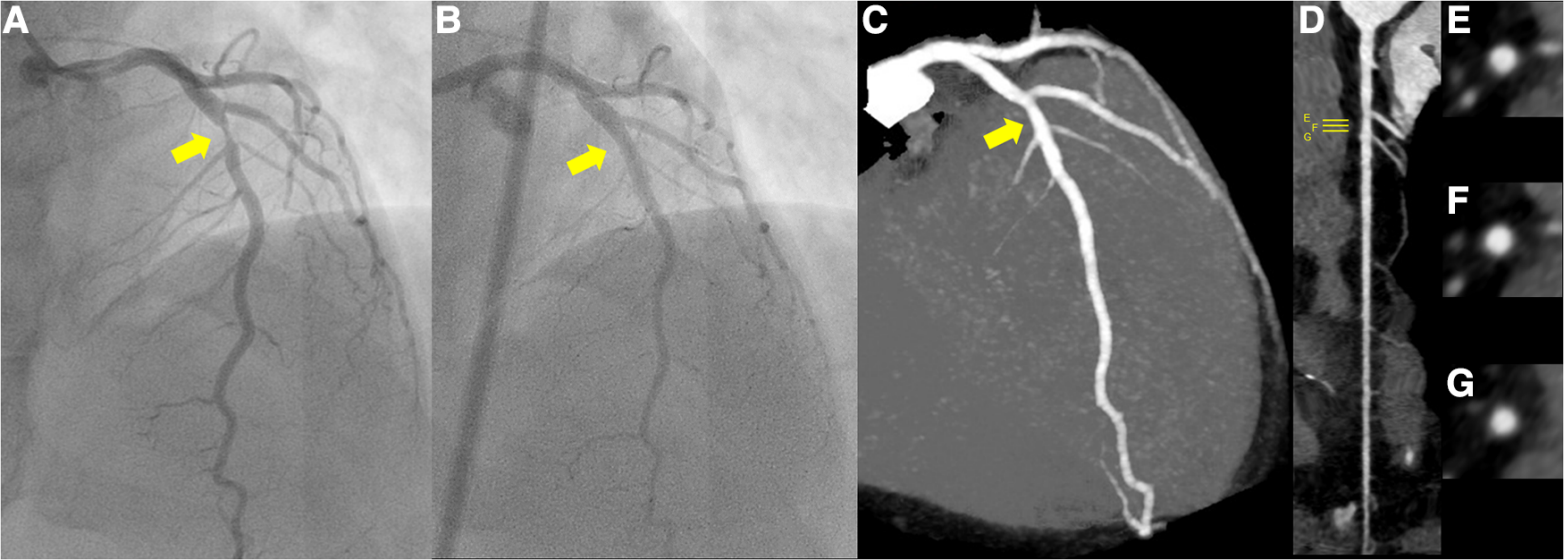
(A) Pre-percutaneous coronary angiogram (PCI) revealing severe stenosis of the left anterior descending coronary artery (yellow arrow). (B) Post-PCI coronary angiogram revealing significant reduction in stenosis of the culprit lesion (yellow arrow). (C–G) Follow-up coronary computed tomography scan at 1 year showed a small soft eccentric plaque with very mild positive remodeling and less than 20% luminal stenosis (yellow arrow; C, maximum intensity projection; D, stretched-curved planar reconstruction; E–G, cross-sections).
